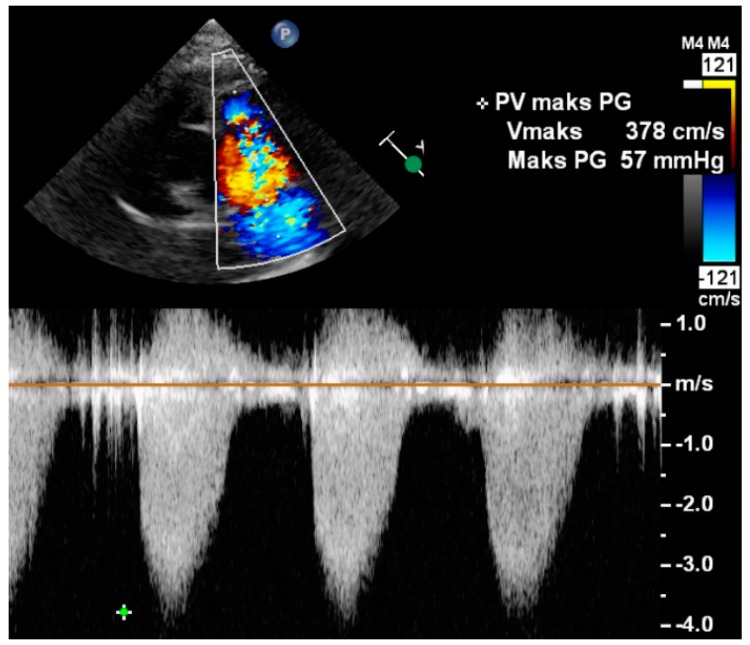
Continuous wave Doppler ultrasound of flow across the pulmonary valve in a patient with pulmonary valve stenosis. Peak pressure gradient (“Maks PG”) shown in upper right corner of the screen was calculated in real-time from peak velocity by using the simplified Bernoulli equation. Image was provided by Klaus Juul (Department of Pediatric Cardiology, Copenhagen University Hospital, Denmark).
